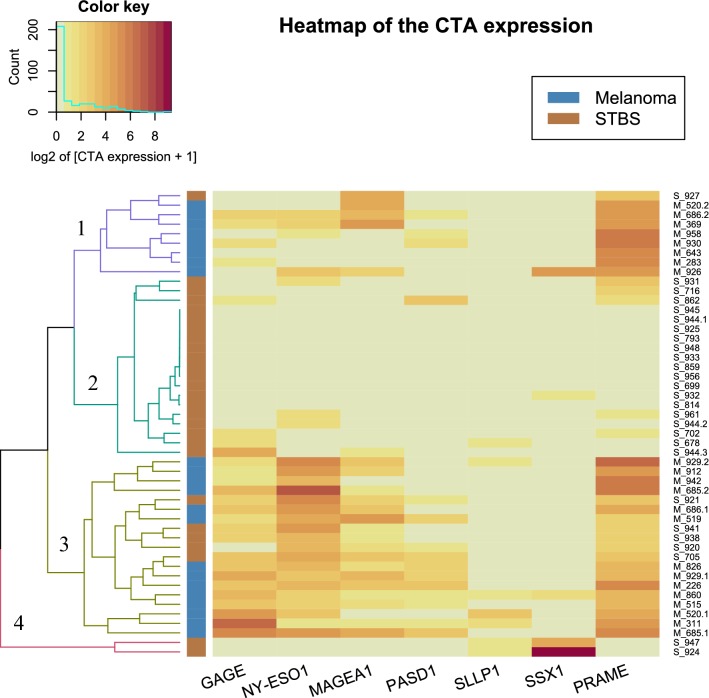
Results of the cluster analysis of the expression of the CTA cells, melanomas, and sarcomas. The intensity of expression is indicated as a log2 of expression of the target gene in relation to the reference gene expression plus one. Melanoma and STBS cultures are marked with blue and orange indicators, respectively
